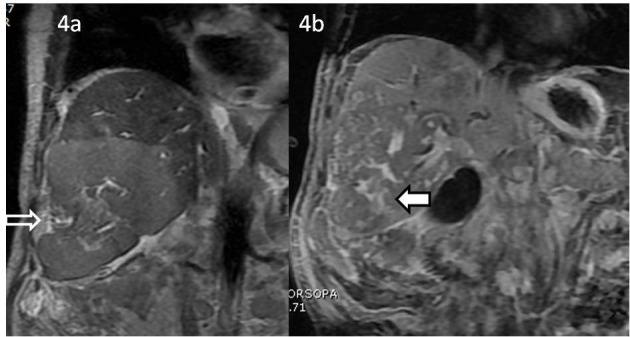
MRI coronal FIESTA (a) and coronal post contrast T1-weighted fat suppressed images after 1 h (b). (a) MRI coronal FIESTA sequence shows striking areas of capsular retraction (white arrow) along the right lobe of liver abutting the mass. (b) MRI post contrast (Gd BOPTA) coronal T1-weighted fat suppressed sequence images acquired after 1 h delay reveal delayed enhancement within few central areas of the mass (bold white arrow with black margins).
